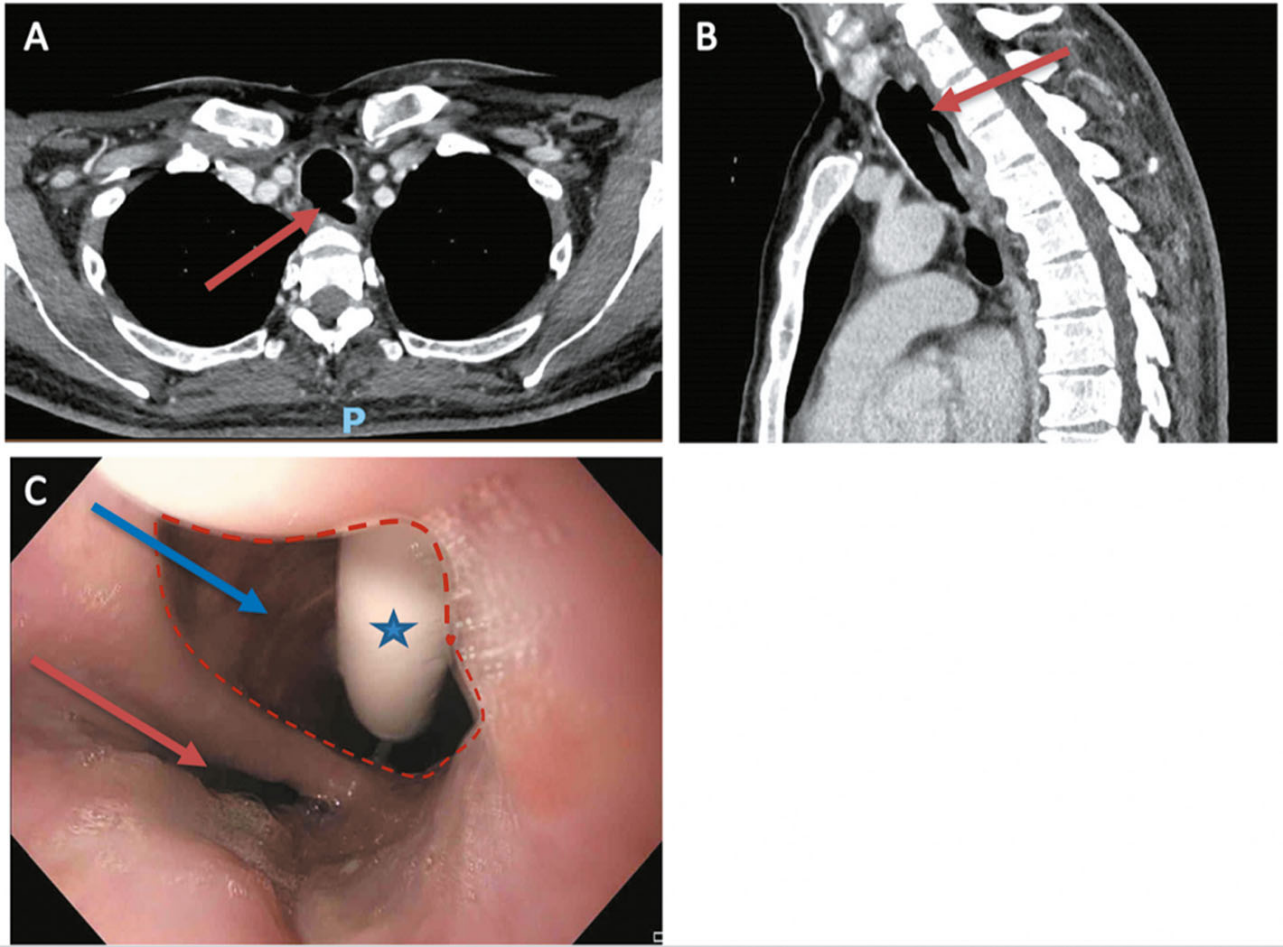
Iatrogenic tracheoesophageal fistula after emergency orotracheal intubation. Computed tomography (CT) images showing a large tracheoesophageal fistula (red arrow) in axial (a) and sagittal plane (b). Endoscopic image (c) of the fistula (red dashed line) between esophageal lumen (blue arrow), with a nasogastric tube inside (blue star), and tracheal lumen (red arrow)
